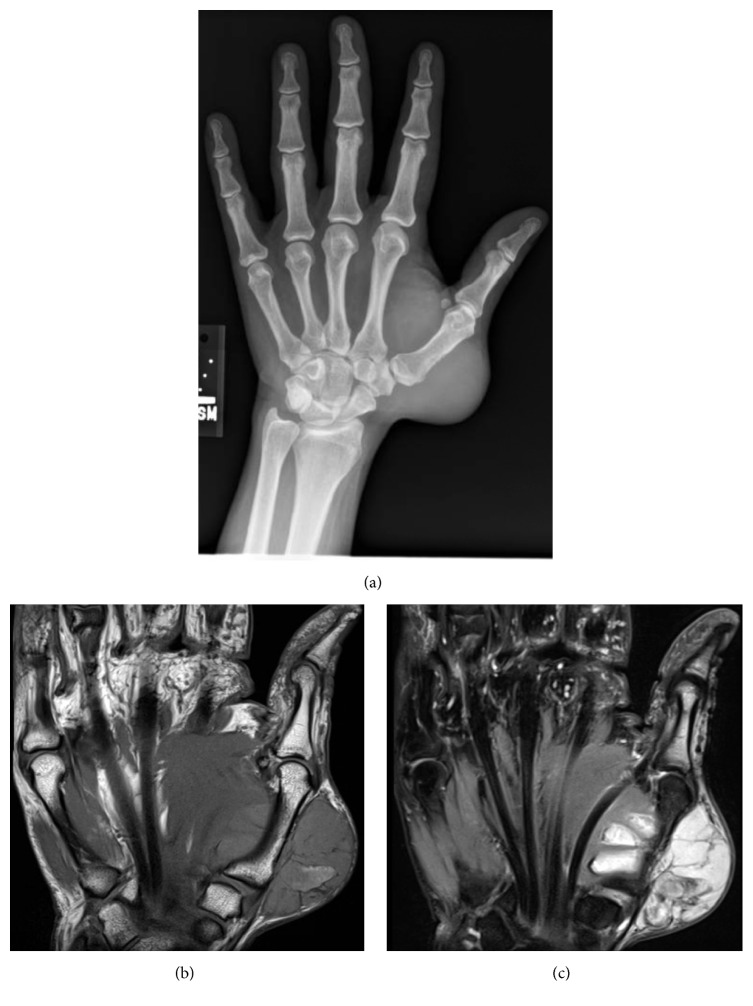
Preoperative imaging. (a) X-rays demonstrating a soft tissue mass with some underlying bony erosion of the first metacarpal. (b) Coronal T1-weighted MRI of the left hand demonstrating envelopment of the first metacarpal. (c) Coronal T2-weighted MRI of the left hand demonstrating involvement of the first metacarpal.
